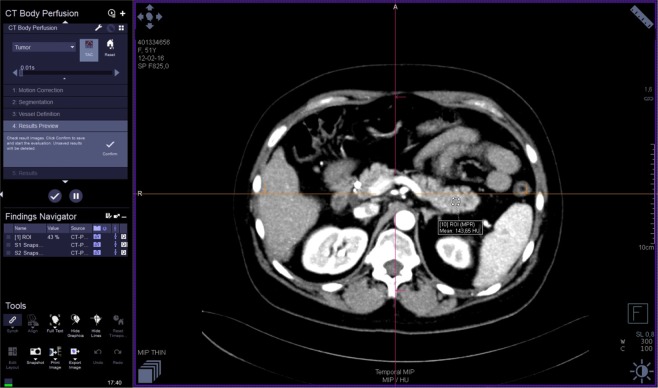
Perfusion CT: ROI positioning in pancreatic tail.
